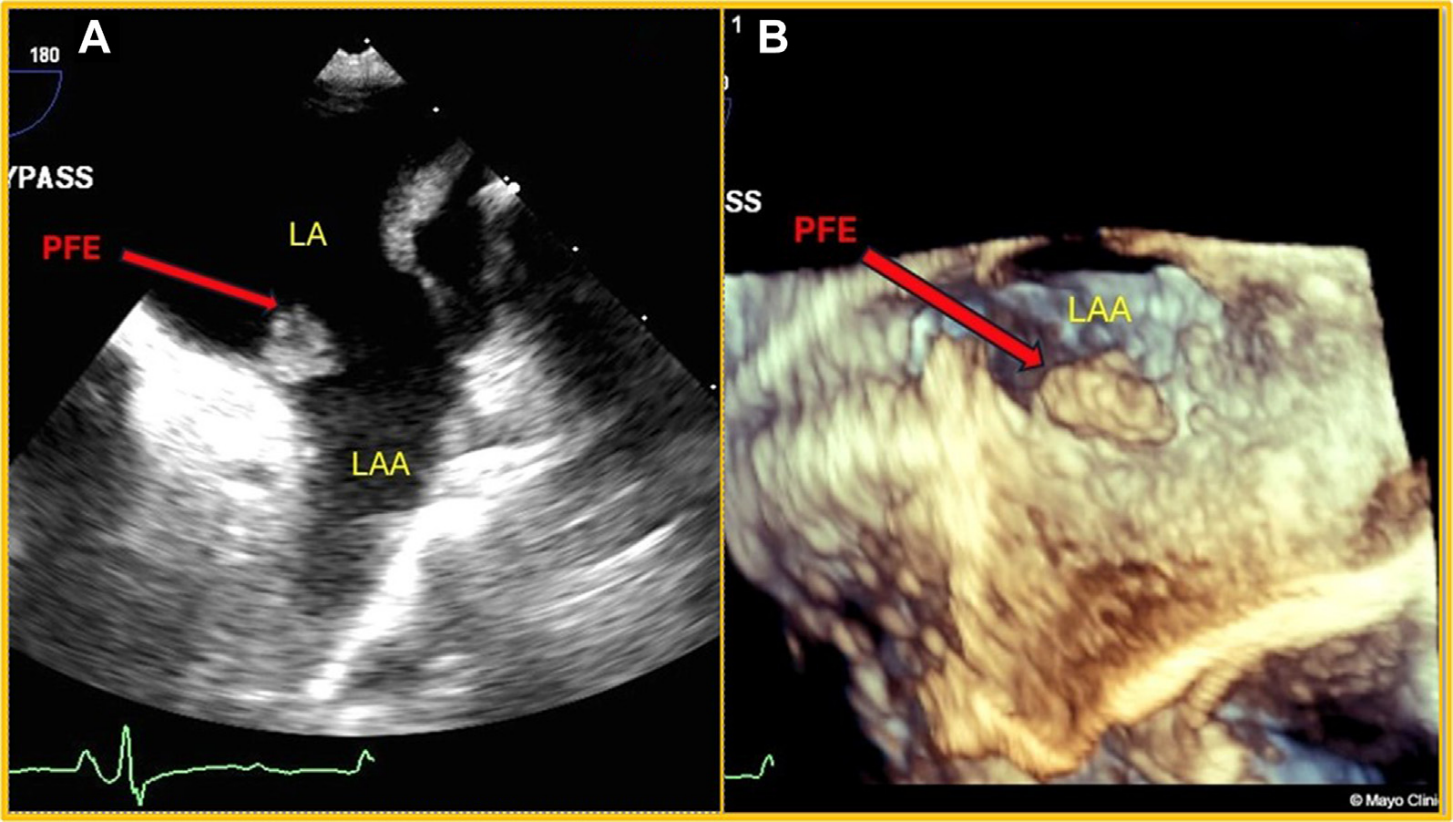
Transesophageal Echo (A) TEE, 2-D TEE midesophageal view of LA with focus on LAA: PFE attached to the orifice of LAA at the junction of the mitral annulus. (B) TEE, 3-D mode-focus on LAA: en-face view of PFE attached to the orifice of LAA. 2-D = 2-dimensional; 3-D = 3-dimensional; LA = left atrium; LAA = left atrial appendage; PFE = papillary fibroelastomas; TEE = transesophageal echocardiography.
